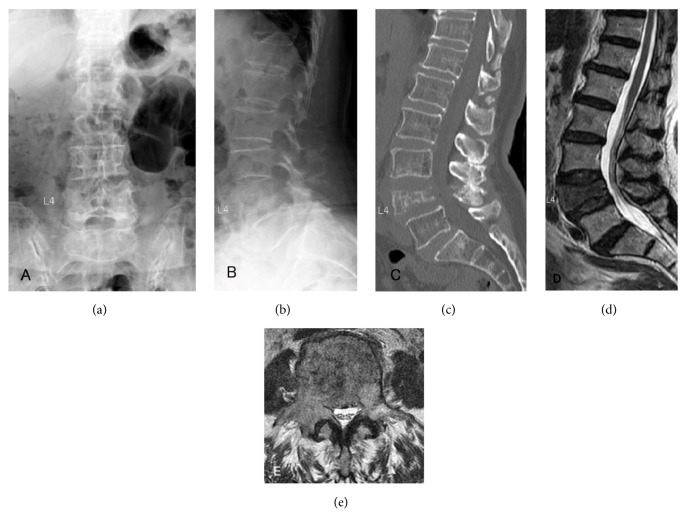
An 80-year-old woman with metastasis of unknown origin to the L4 lumbar vertebrae. The preoperative ASIA classification was E. The modified Tokuhashi score was 11. (a) Posteroanterior view on preoperative radiography. (b) Lateral view on preoperative radiography. (c) Sagittal view on preoperative plain CT. (d) Sagittal view on preoperative T2-weighted MRI. (e) Axial view at the L4 lumbar vertebrae on preoperative T2-weighted MRI.
